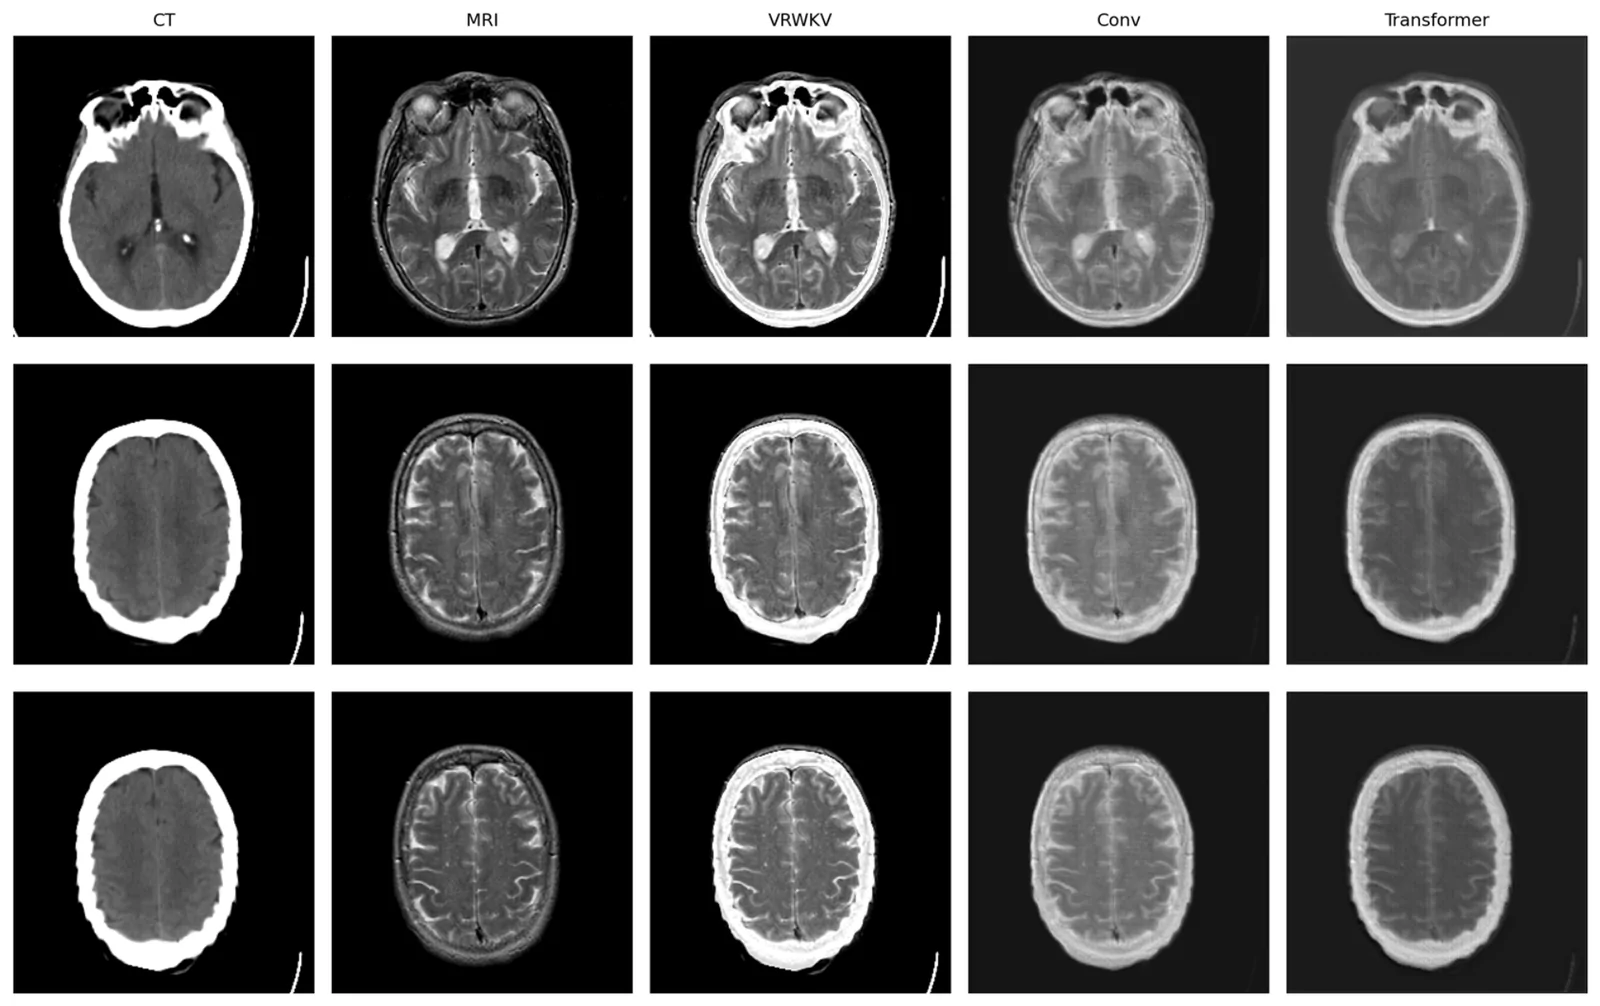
Qualitative comparison of fusion results among VRWKV, Conv, and Transformer configurations on a representative CT–MRI image pair. This ablation visualization demonstrates that replacing the VRWKV block with standard convolutions leads to a loss of global structural coherence, while the Transformer configuration introduces noticeable artifacts due to optimization difficulties under limited data. The full VRWKV configuration achieves the optimal balance, preserving both fine local details and global anatomical consistency.
